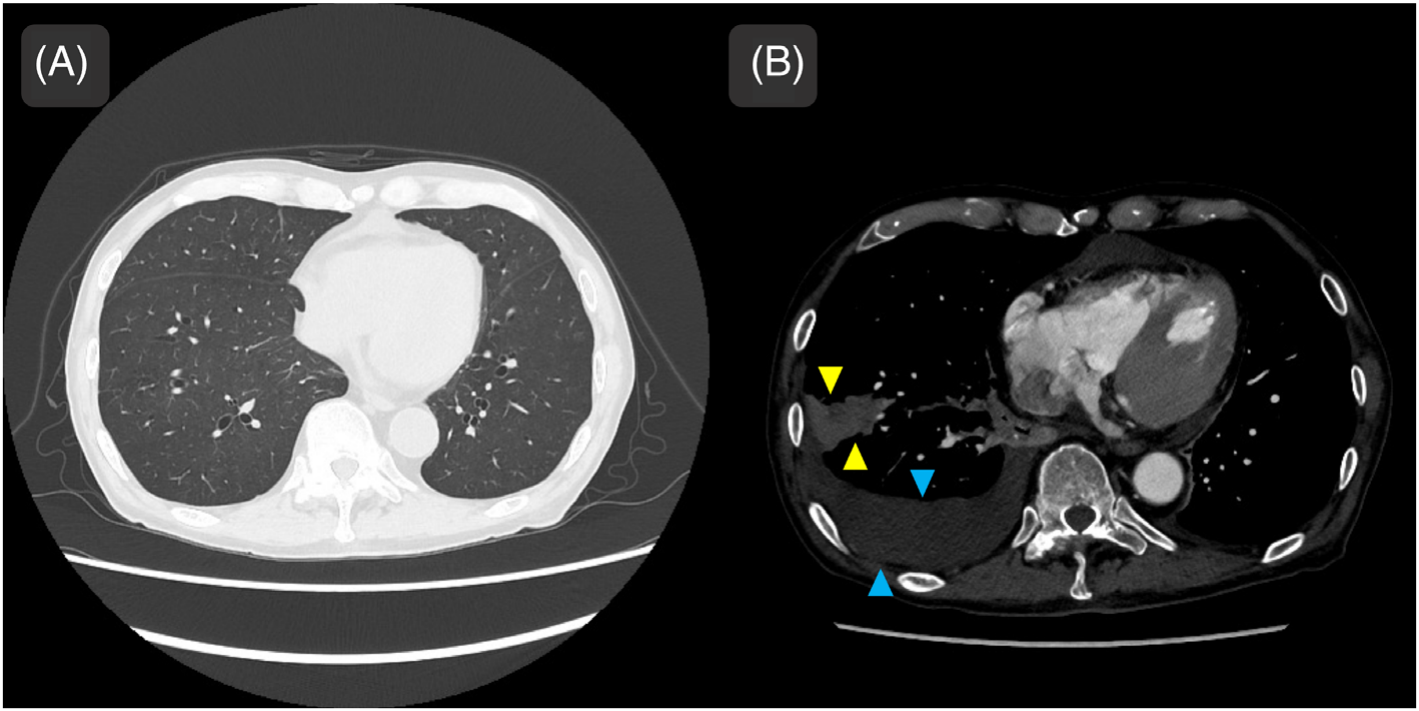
Imaging findings. (A) Chest CT shows bilateral diffuse ground‐glass opacities. (B) Contrast‐enhanced chest CT after transbronchial lung cryobiopsy shows a wedge‐shaped infiltrating shadow (yellow arrow head indicated) in the right lower lobe and a right hemothorax (blue arrow head indicated). There is no evidence of active haemorrhage. CT, computed tomography
